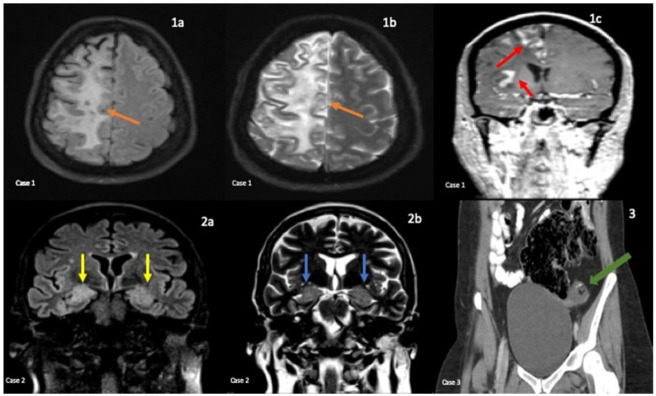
CASE 1: Axial FLAIR (1a), Coronal T2 (1b), and post contrast coronal T1 RAGE (1c) sequences. Multifocal areas of confluent subcortical T2/FLAIR hyperintensity (orange) with overlying gyriform cortical enhancement (red) involving the right frontal, parietal, and occipital lobes. CASE 2: Coronal FLAIR (2a) and T2 (2b) sequences. Symmetric T2 (blue) and FLAIR (yellow) hyperintensity with mild swelling in the mesial temporal lobes involving the amygdala and hippocampi. CASE 3: Coronal CT of the pelvis with IV and oral contrast (3). Left ovarian mass containing fat and calcification consistent with a teratoma (green).
